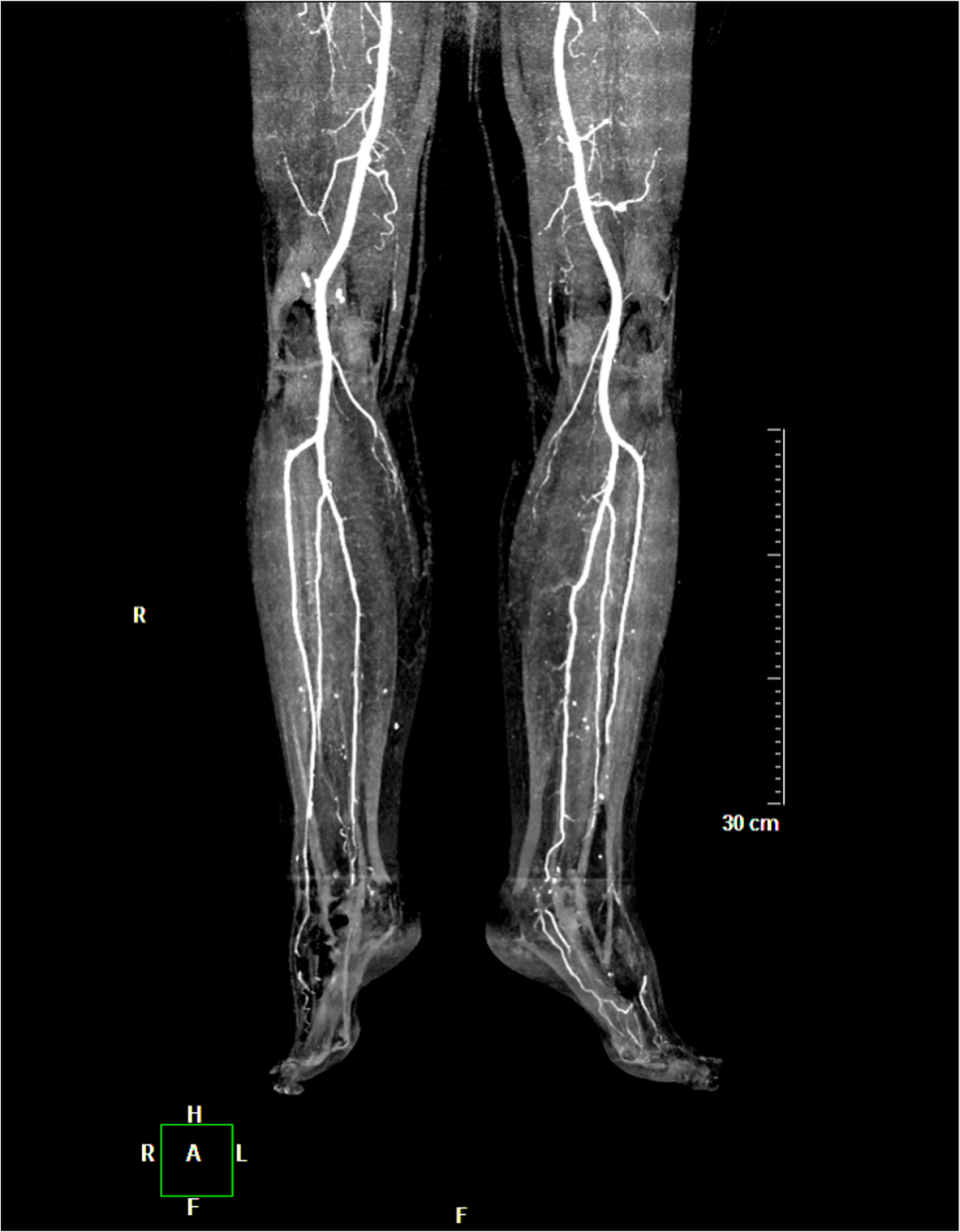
Coronal maximum intensity projection (MIP) of lower extremity CT angiography ruling out any significant arterial stenosis
